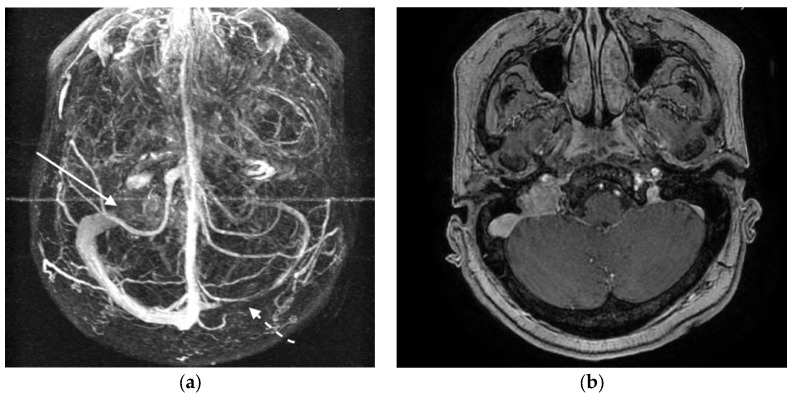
(a,b) Contrast-enhanced MRI and magnetic resonance venography demonstrating hypoplasia of the right transverse sinus (dotted arrow) and tumor invasion of the left jugular bulb (continuous arrow).
